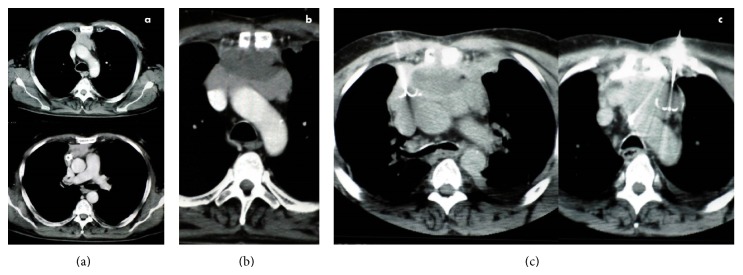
CT scans depicting the preoperative aspect of the lesion (a), the necrosis obtained after surgery and RFA (b), and the positions of the electrodes during percutaneous RFA performed after surgery (c).
